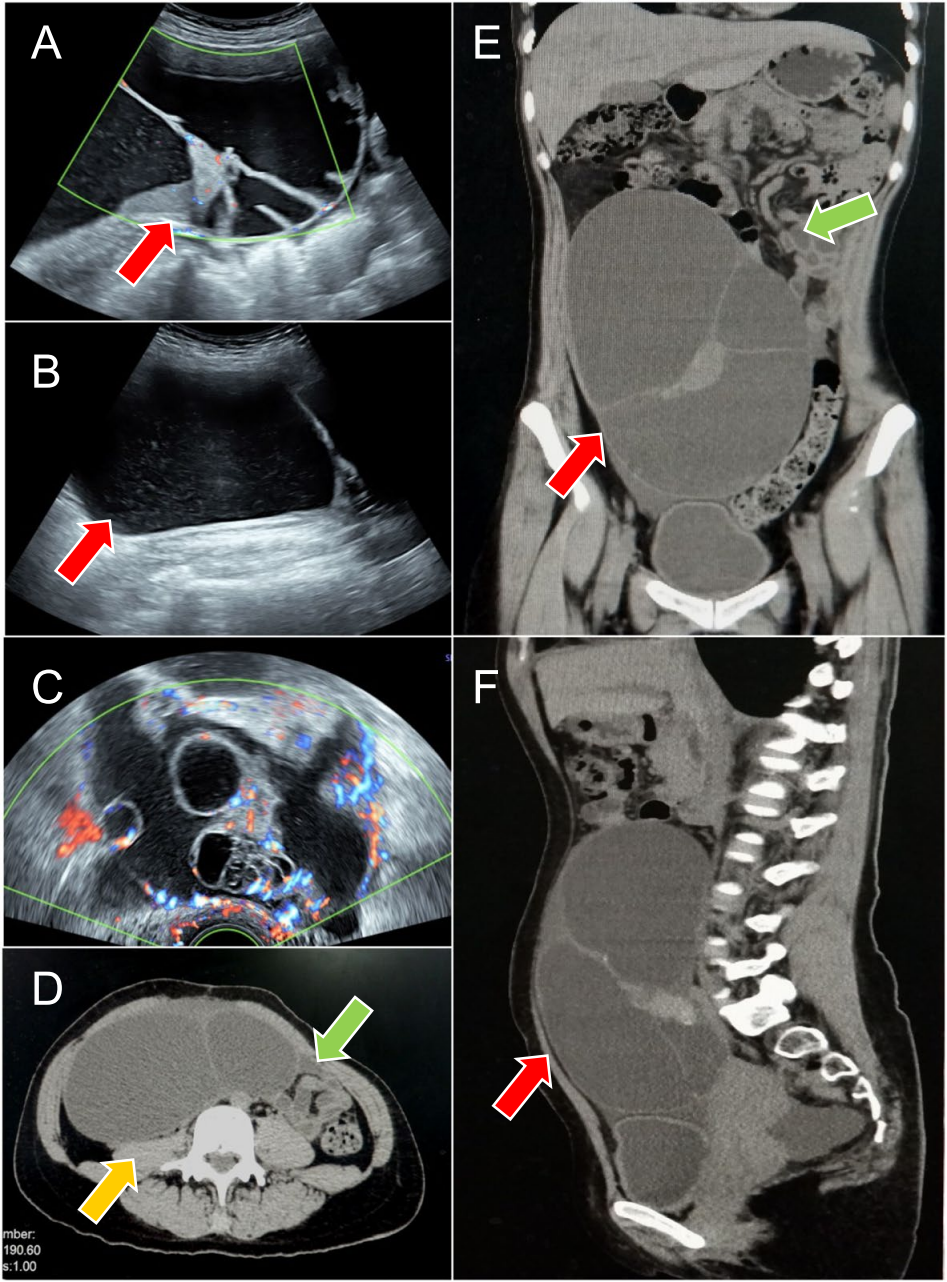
The red arrows represent pelvic and abdominal masses, this yellow arrow represents the compressed renal pelvis and the right ureter, and the green arrows represent the compressed intestine. Figures A-C are gynecological ultrasound images. Figures A and B show a giant fluid-dominated mass from the suprapubic area between the umbilicus and the glabella, reaching the anterior axillary line on both sides, with a size of about 28.6×14.2 cm. Figure C: color Doppler ultrasound showed no significant abnormal blood flow signal. Figure D-F: the size of the lesion was about 15.0×7.1×20.2 cm, with no significant enhancement of the cystic component and more uniform enhancement of the solid component after enhancement
